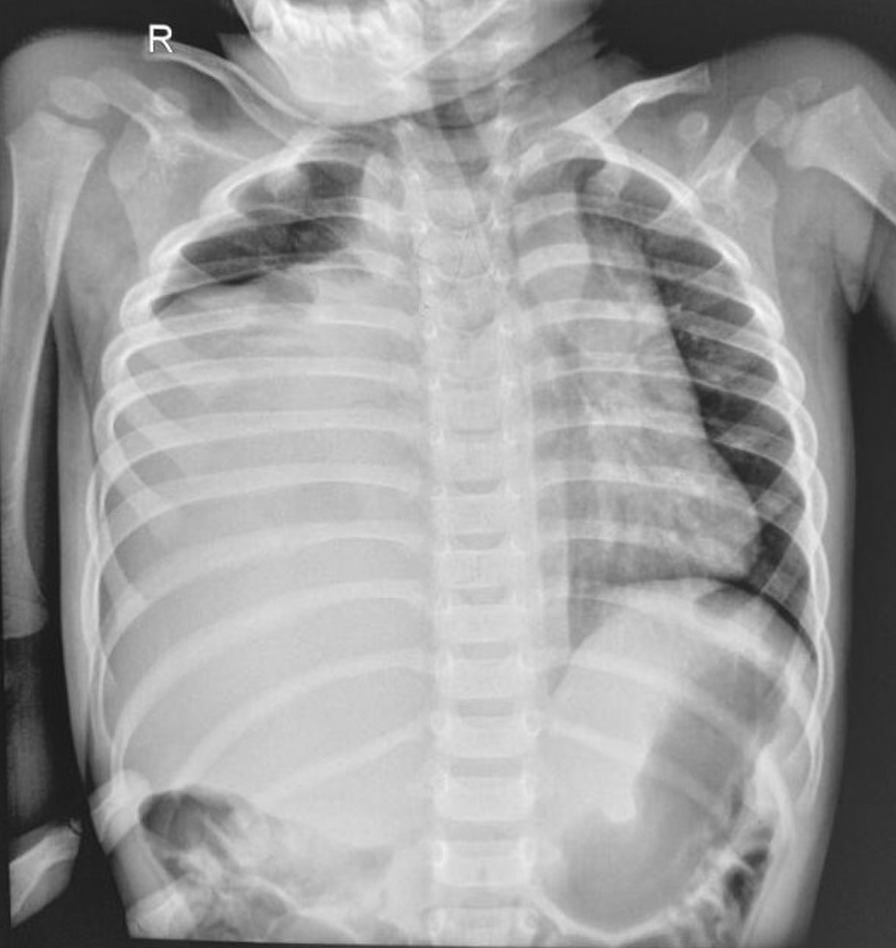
Chest X-ray showing well-demarcated opacity in the lower and mid zones of the right hemithorax with shifting of the trachea to the left side
